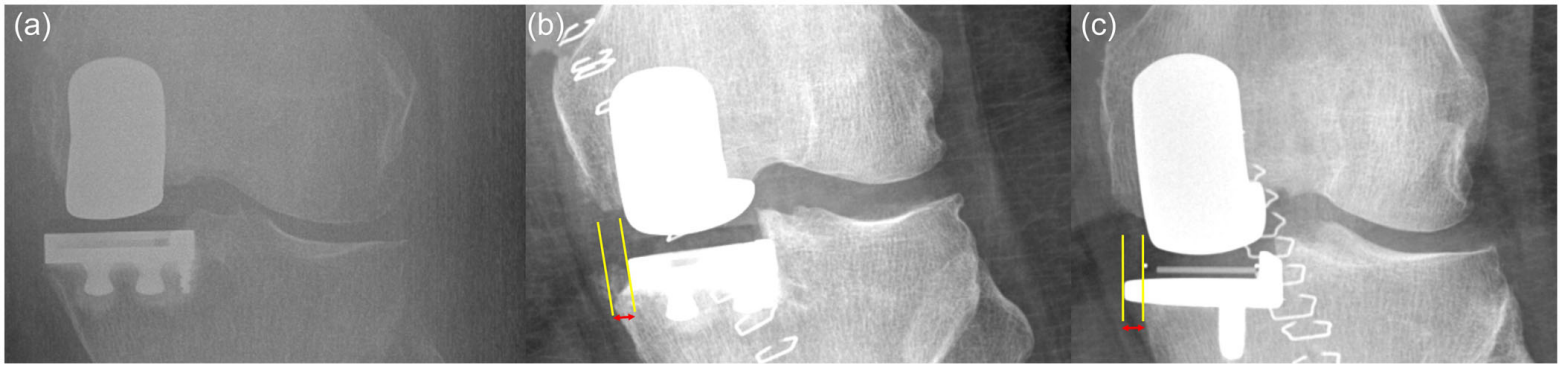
Radiographic depiction of a unicompartmental tibial component in a fit (a), under‐hang (b) and over‐hang (c) positions.
